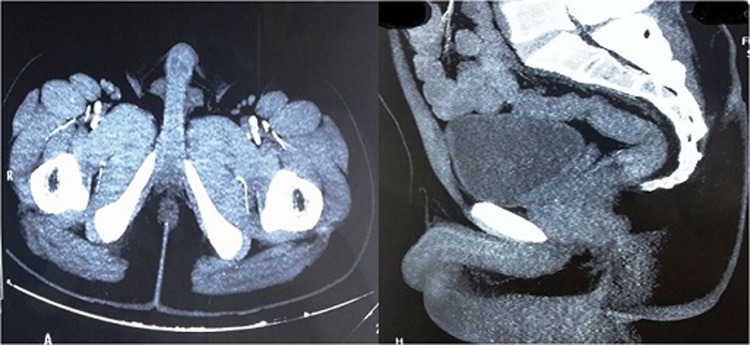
CT angiography images (axial, sagital cuts) showing resolution of pseudoaneurysm after USG-guided compression technique.
